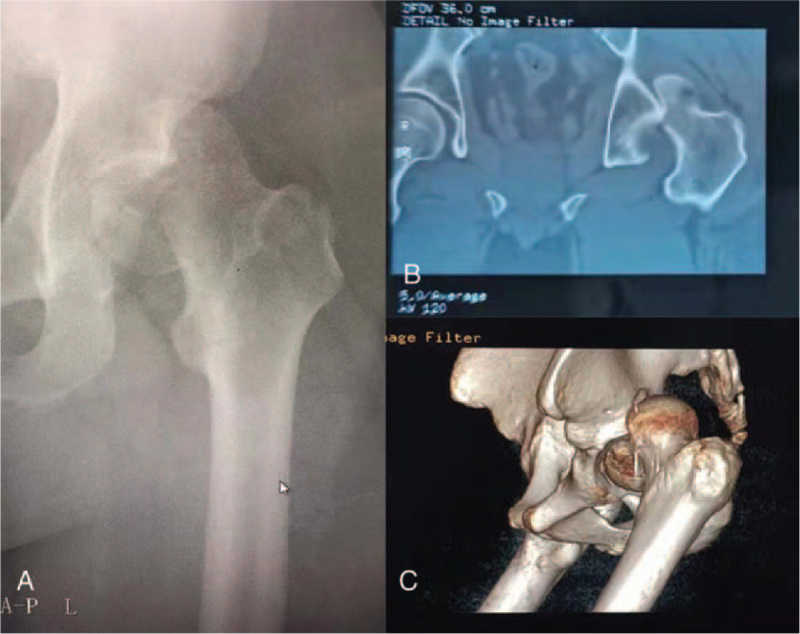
X-ray as well as computed tomography (CT) examination before closed reduction of hip dislocation. (A) Anteroposterior x-ray image of the left hip. (B) Coronal CT scan showing femoral head fractures and dislocation of hip joint. (C) 3-Dimensional CT of hip showing femoral head fractures and posterior dislocation of hip joint.
